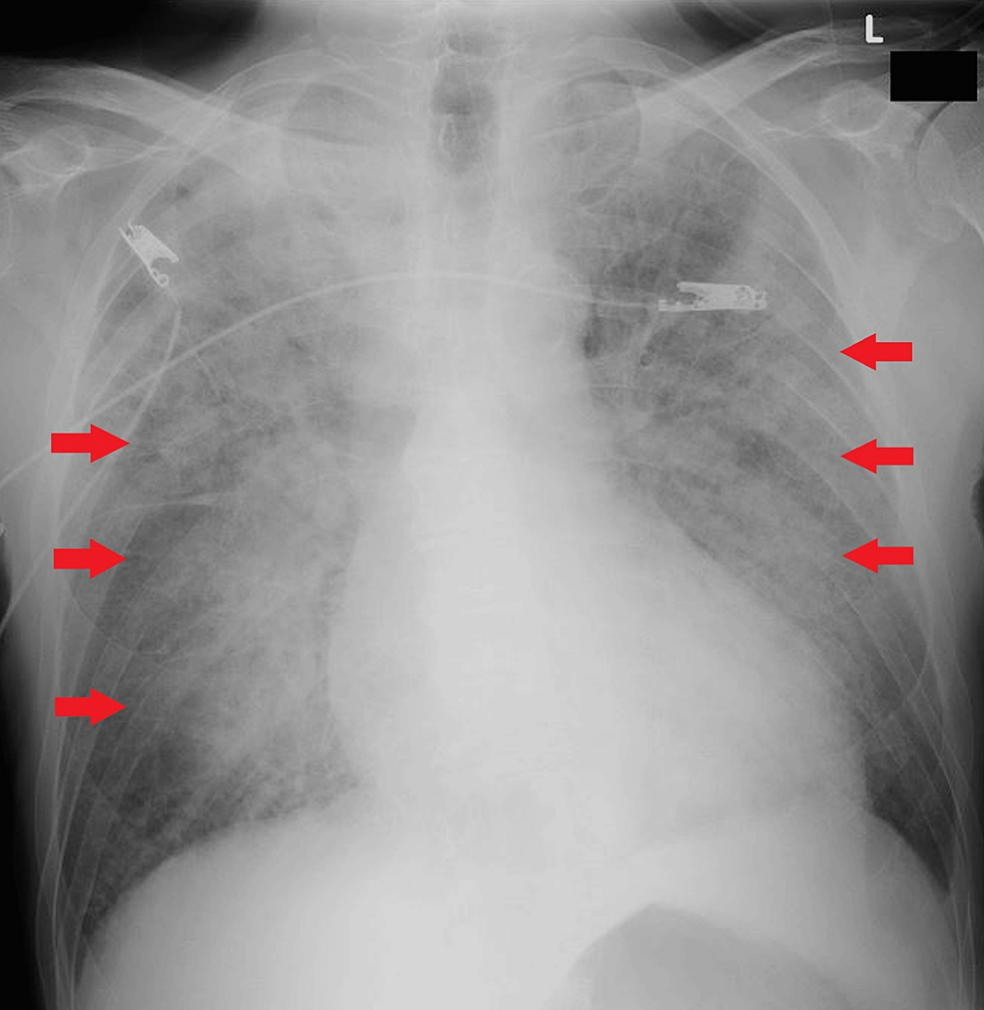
Chest X-ray on hospital admission in case 2. A typical butterfly shadow is noted (red arrows).
